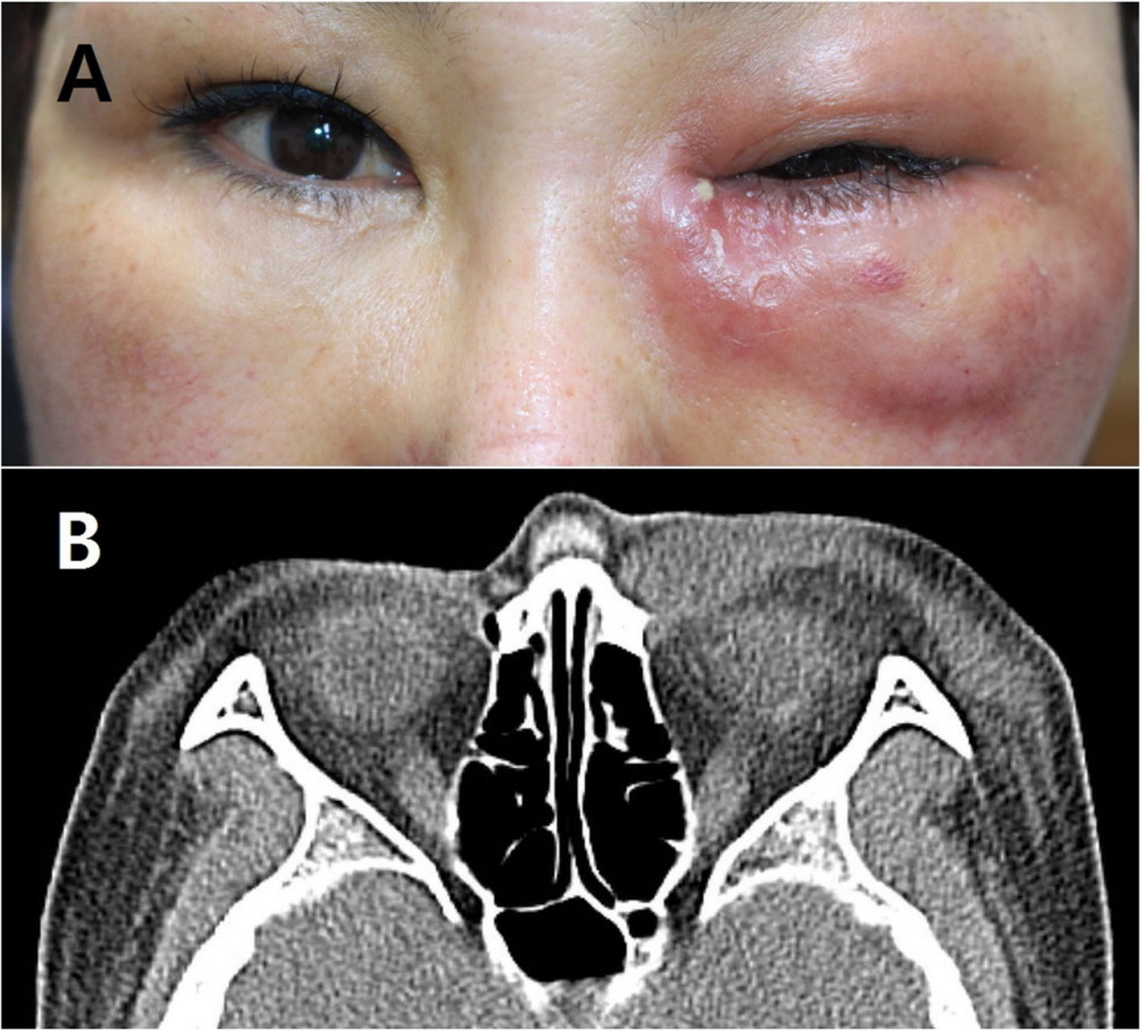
A External photograph showing swelling and erythema of the left eyelid, B facial computed tomography image showing soft tissue swelling in the left periorbital area
